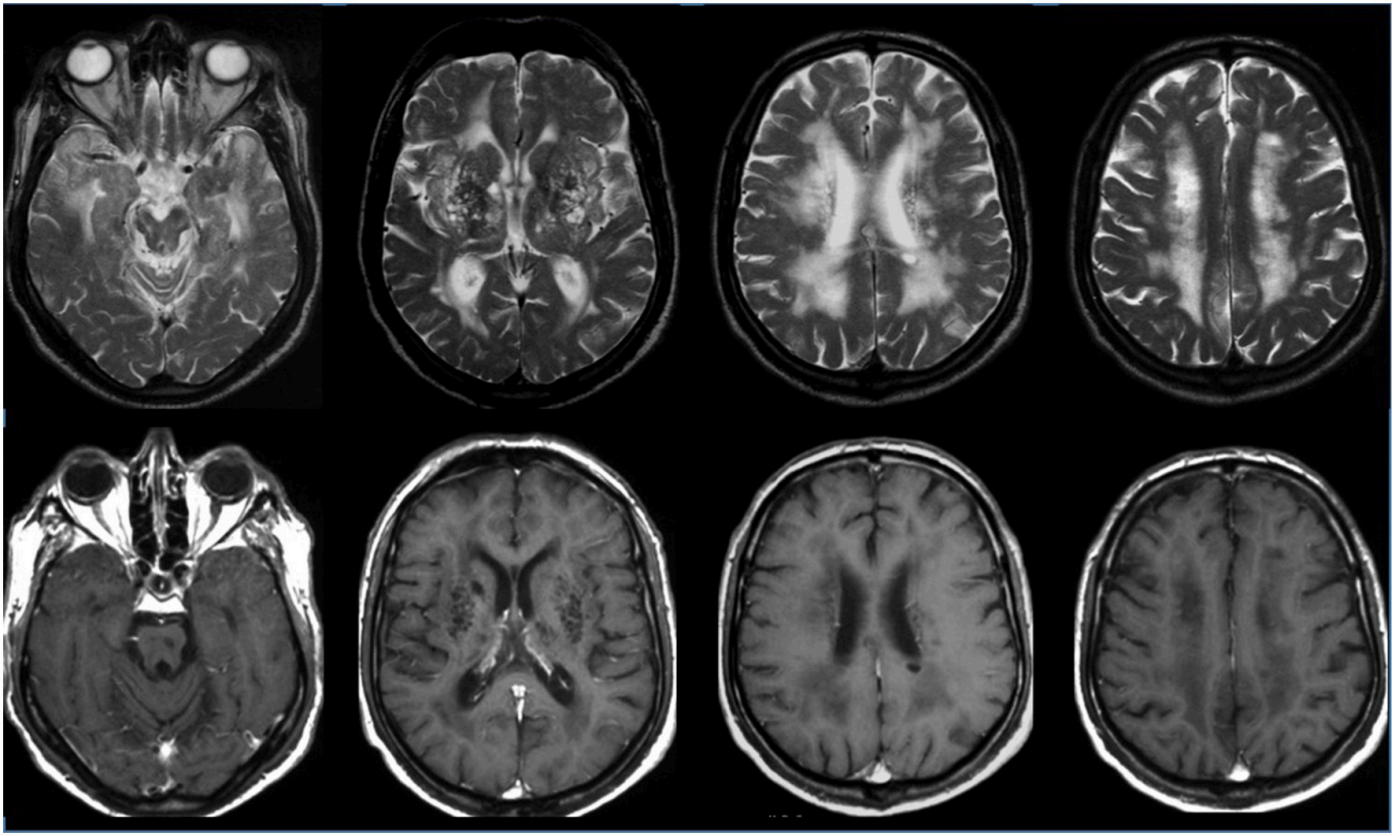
Neuroimaging of cerebrovascular disease in the aging brain. The figure shows a brain MRI study of a patient with risk factors affected by cognitive disorders. The images above (T2-weighted axial slices) and below (T1-weighted axial slices, after contrast enhancement) show diffuse, punctate deep white matter foci, hyperintense T2-weighted images, with a low signal intensity on T1-weighted images and without contrast enhancement, suggesting cerebral small vessel vascular disease.
